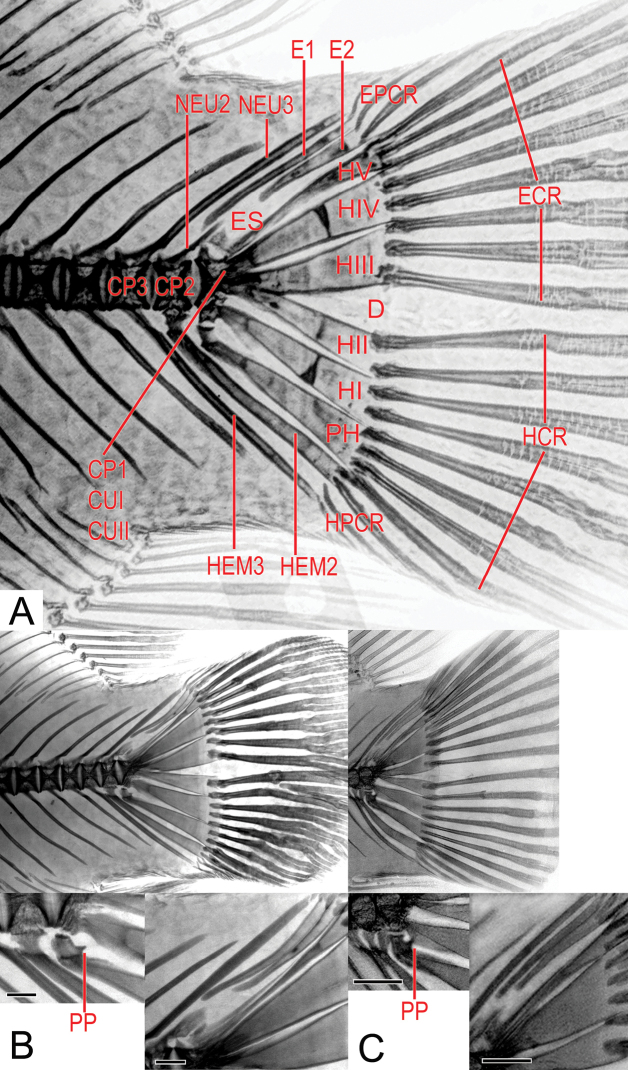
Radiographs of the caudal skeleton in *Astronotus* species showing magnified details A*A.mikoljii* sp. nov. (MHNLS 24059, 205.5 mm SL) B*A.crassipinnis*INPA-ICT 33889 (204.3 mm SL) C*A.ocellatus*USNM 284442 (79.6 mm SL). Abbreviations: hypurapophysis (PP) of parahypural bone (PH), epurals (E1 – E2) and neural spine (NEU2, diastema (D), preural centrum (CP), epaxial caudal rays (ECR), hypaxial caudal rays (HCR), epaxial procurrent caudal rays (EPCR) hypaxial procurrent caudal rays (HPCR). Scale bars: 2 mm.
